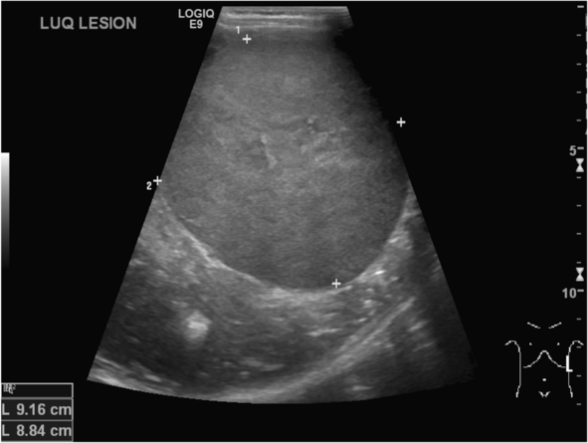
Ultrasound abdomen of a 4 year old boy with a left-sided Wilms tumour, presenting here unusually as a solid uniform mass.
